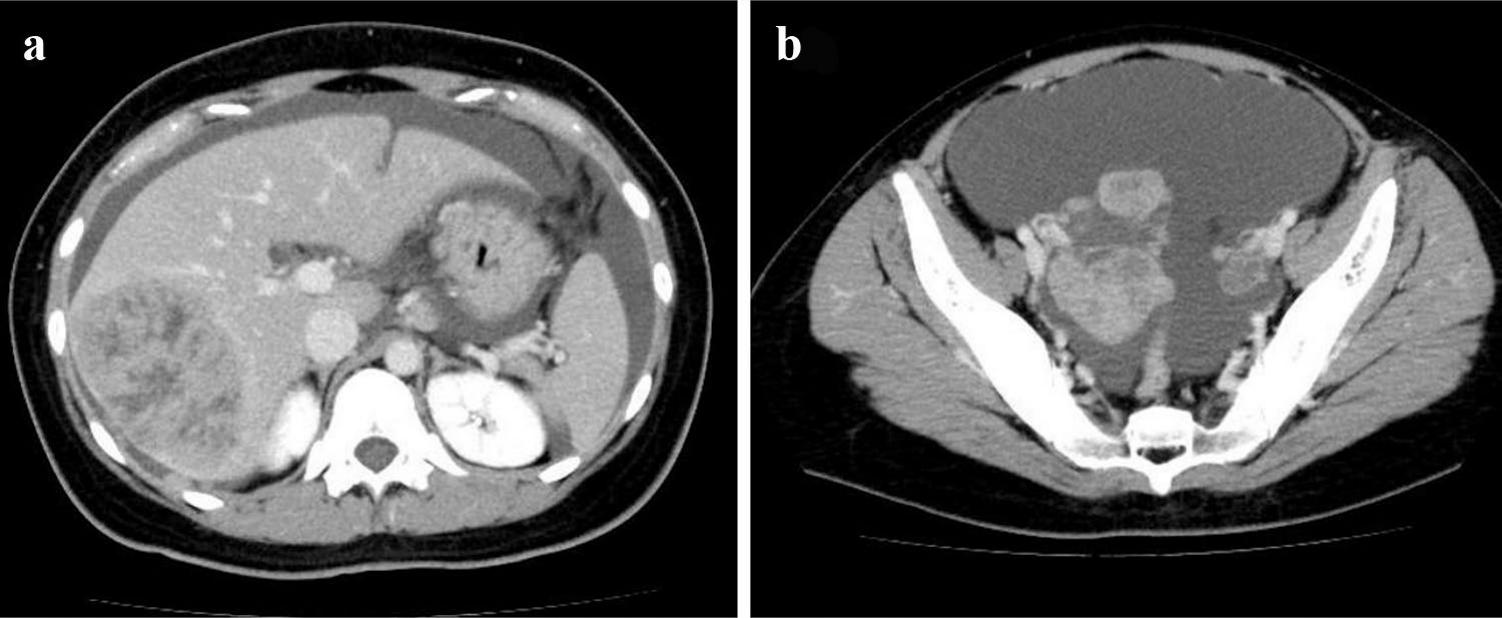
Abdominal CT at presentation at our hospital. a Abdominal CT revealed huge hepatic tumor in the right lobe. b A moderate amount of ascites, and a right ovarian tumor was also seen
